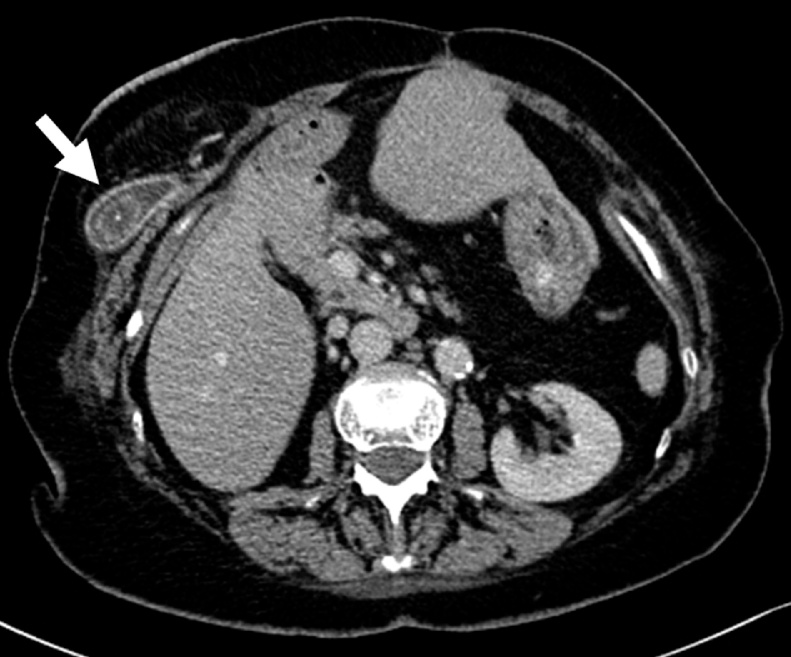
Abdominal CT scan. Gallbladder herniated into the parastomal hernia (arrow).
